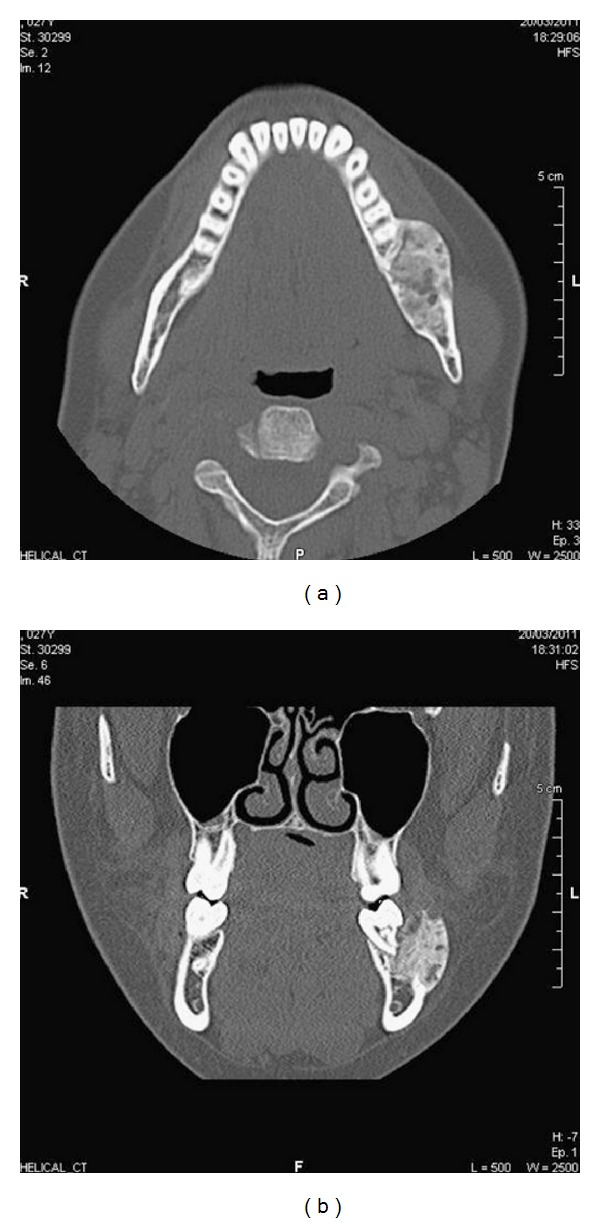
(a) Axial CT of the mandible showing the brown tumour as a mass of the left hemimandible. (b) Coronal CT scan of the brown tumour with well-defined radiolucent osteolytic lesions encircling the roots of teeth.
